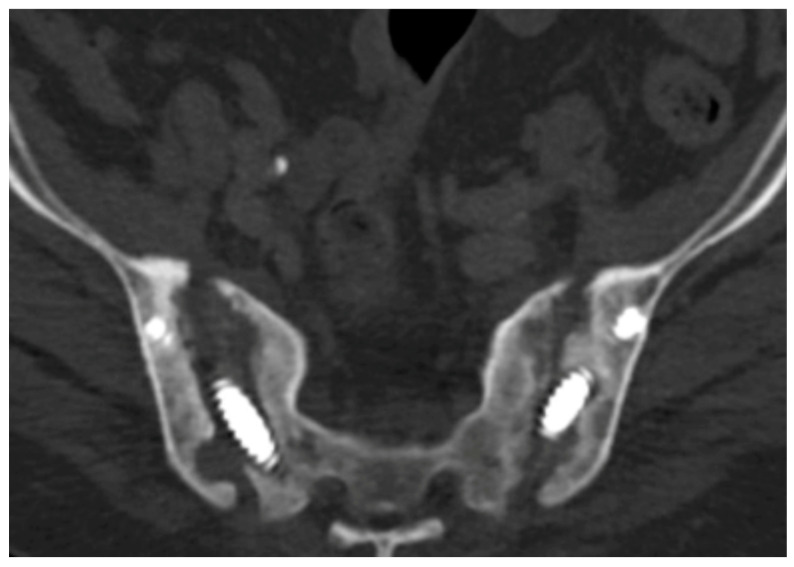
Axial CT view of the pelvis showing bilateral SI joint erosion and previous hardware.
